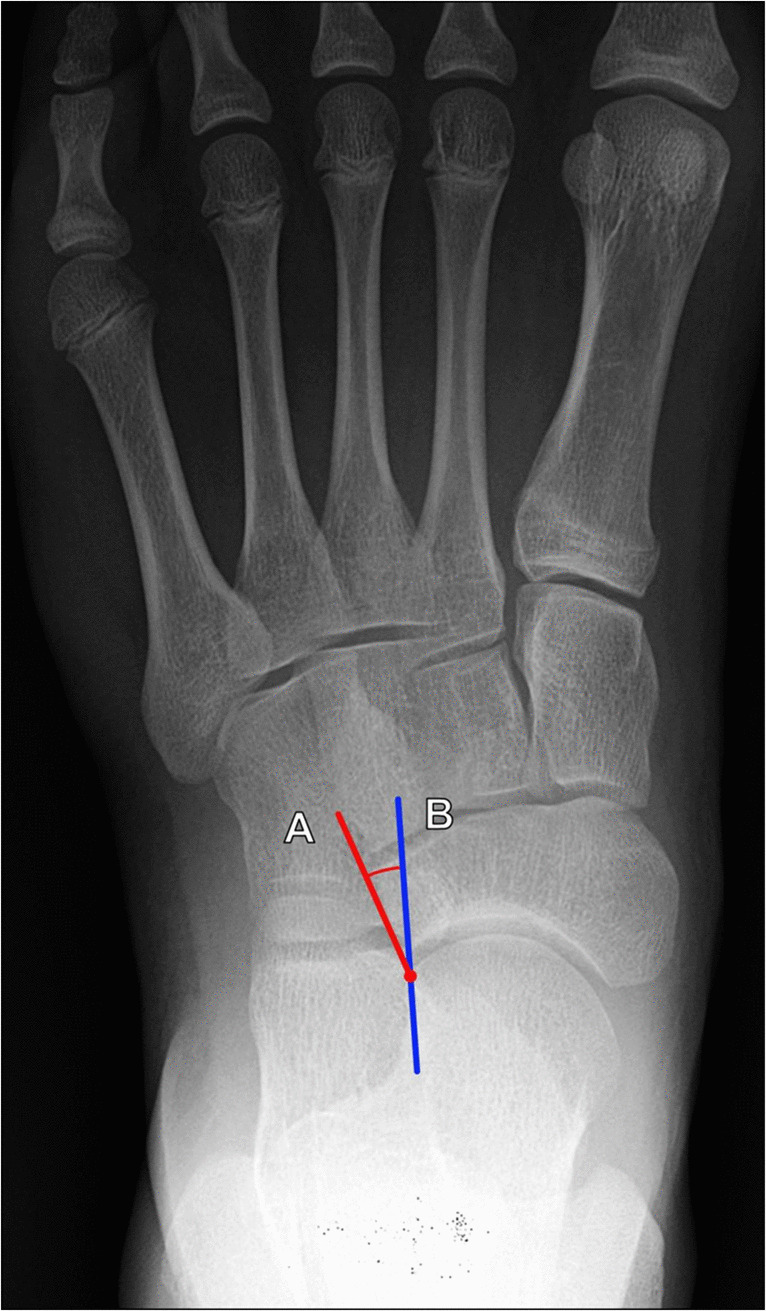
Measurement of talonavicular incongruency angle on DP weightbearing radiograph of the foot. The angle is formed from a line connecting the lateral aspects of the talar and navicular articular surfaces (A, red line) and a line connecting the lateral margin of the talar neck at its narrowest segment and the lateral talar articular surface (B, blue line). A larger incongruency angle indicates greater forefoot abduction and more severe pes planus, and a measured value > 45° reflects stage IIB flatfoot deformity
